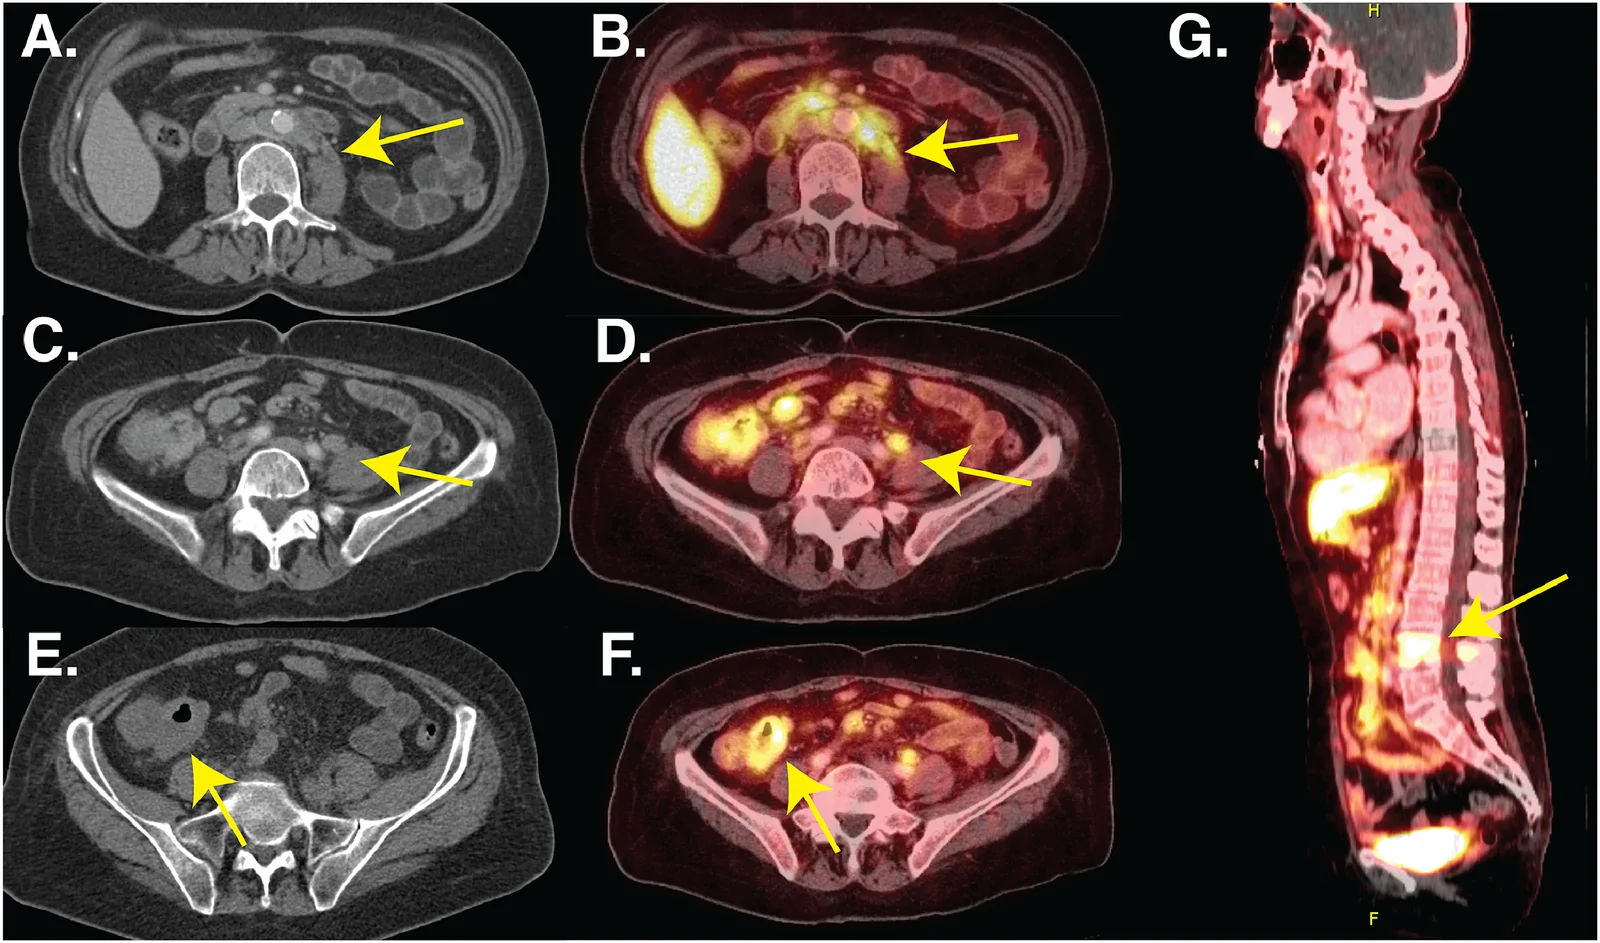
^68^Ga-DOTATATE PET/CT Findings. Axial CT (A, C, E) with fused PET/CT (B, D, F) and sagittal projection of PET/CT (G). (A, B) Left periaortic lymphadenopathy measuring up to 21 mm with moderate DOTATATE activity (SUV_max_ 6.3, yellow arrow). (C, D) DOTATATE-avid left common iliac lymph node (SUV_max_ 5.1, yellow arrow), representing biopsy-confirmed follicular lymphoma. (E, F) Terminal ileal wall thickening with moderate DOTATATE uptake (SUV_max_ 6.3, yellow arrow), representing active Crohn's disease. (G) Sagittal projection demonstrating anatomically distributed DOTATATE uptake, including L4 vertebral body with heterogeneous moderate DOTATATE activity (SUV_max_ 7.7, yellow arrow).
